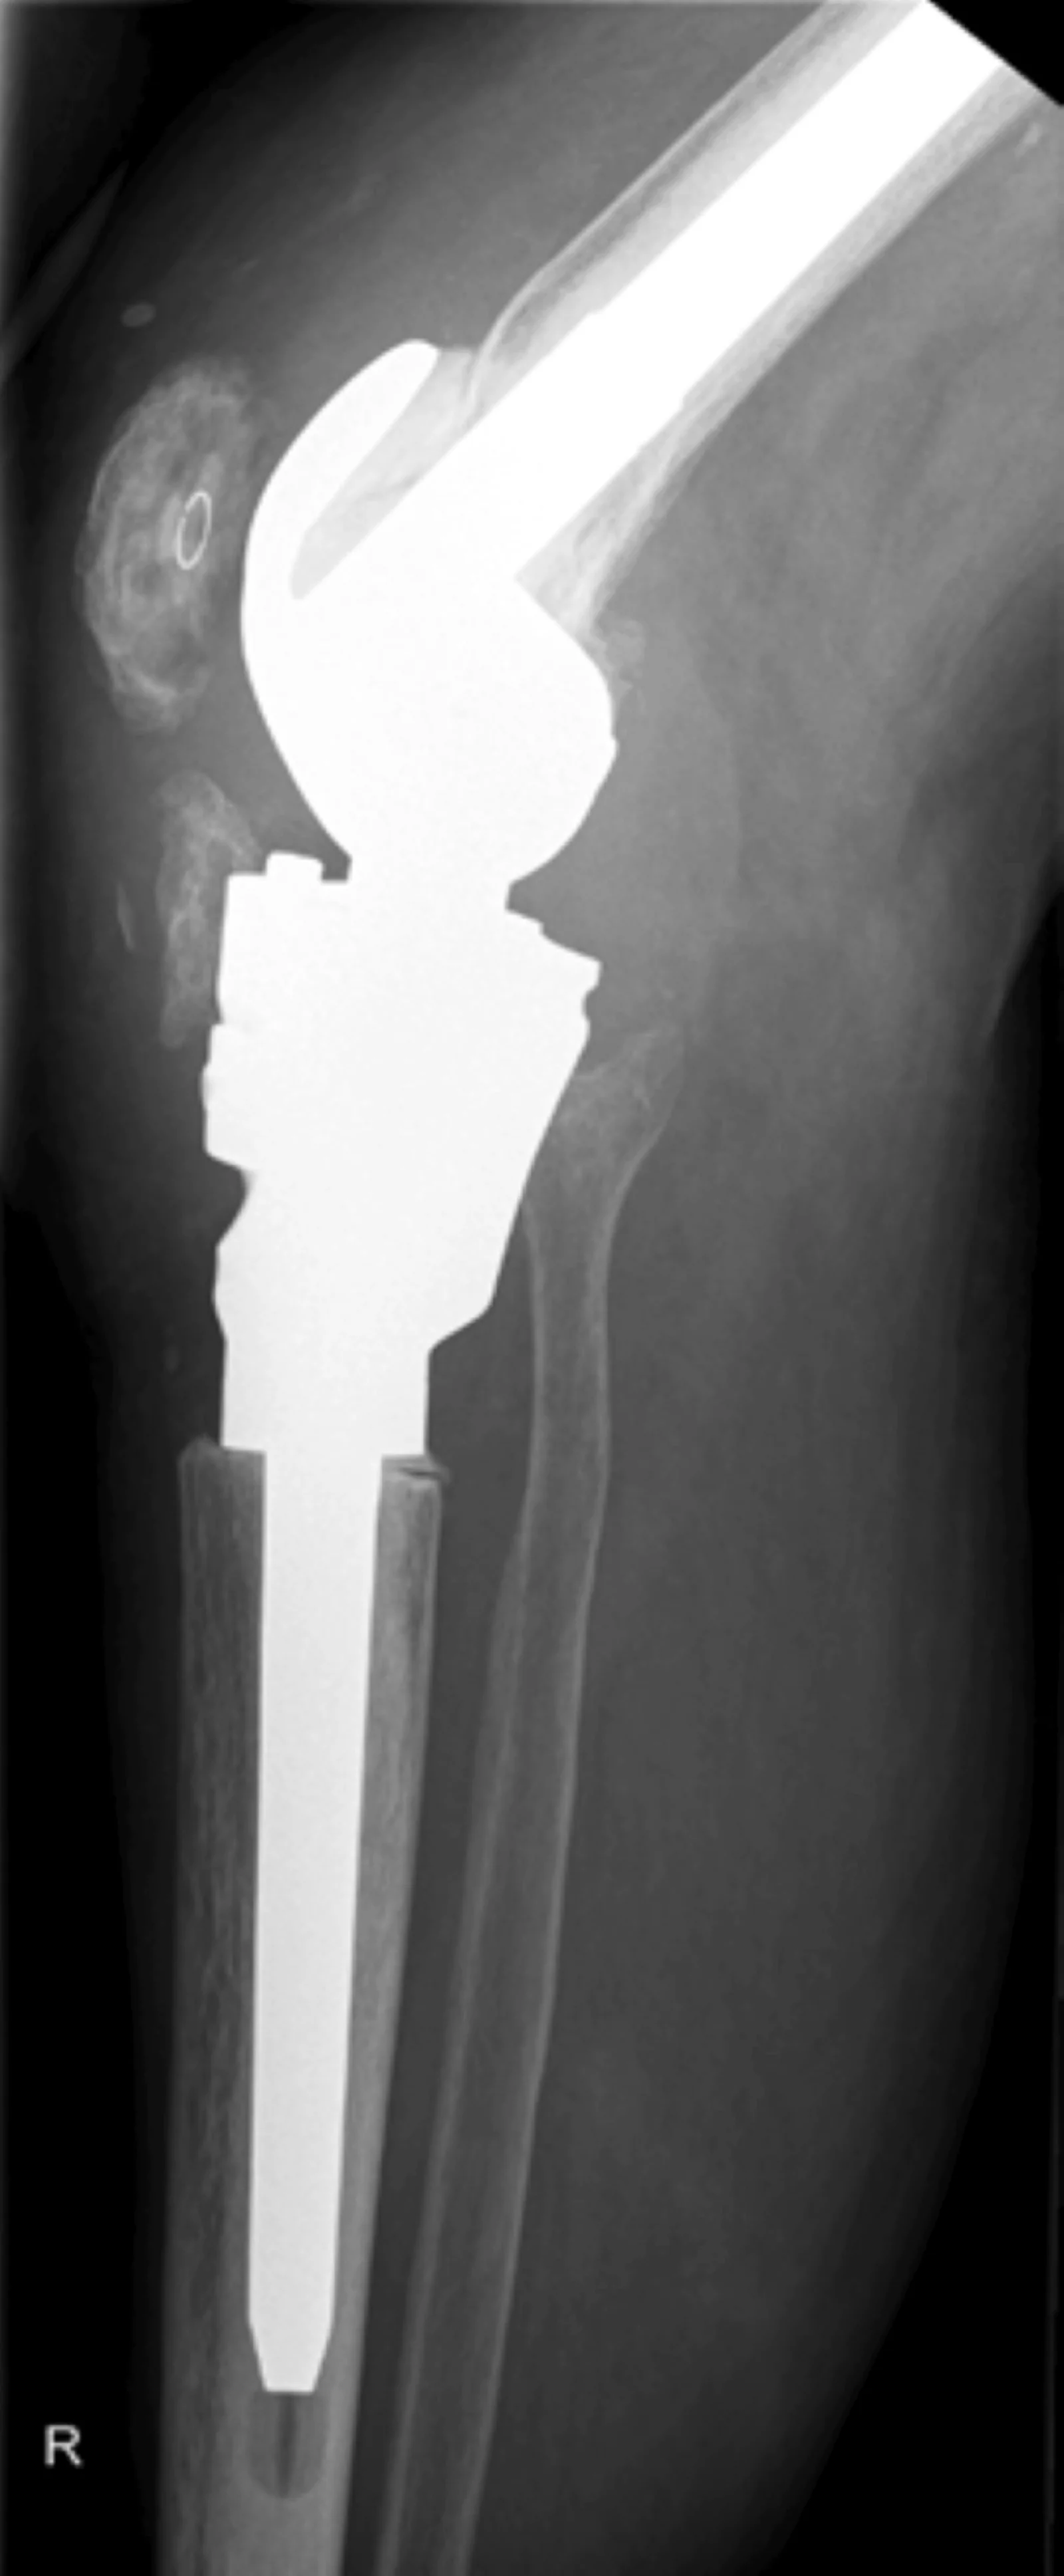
Lateral radiograph of the right knee one year postoperatively showing appropriate implant positioning and no complications after revision arthroplasty.
